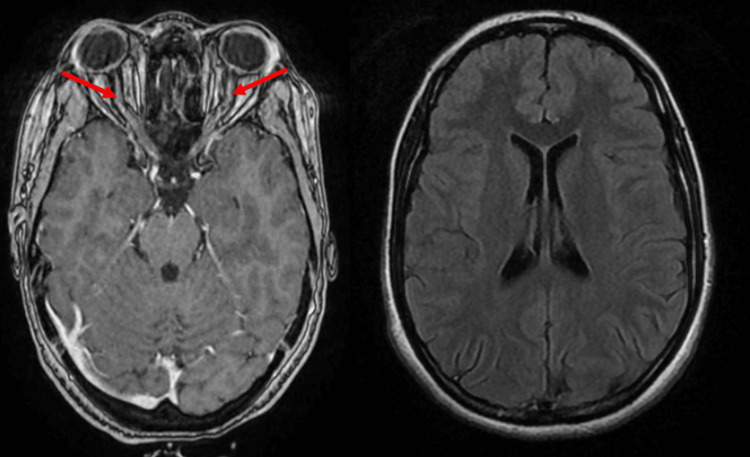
Cerebral MRI showing bilateral optic neuritis with gadolinium enhancement
